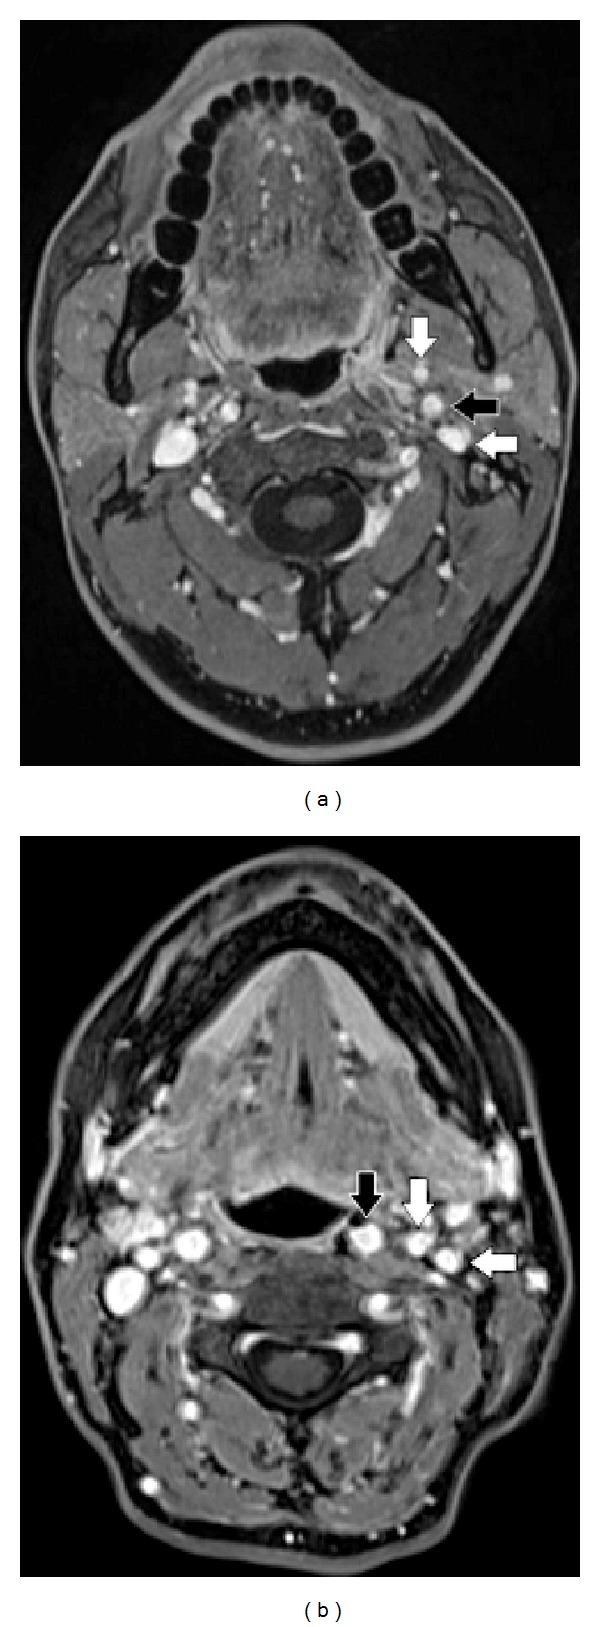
Patient 1 and patient 2, respectively: T1 weighted enhanced MRI scan. The internal carotid artery (black arrow), the external carotid artery (vertical white arrow), and the internal jugular vein (horizontal white arrow) are replaced in their original position with no evidence of disease.
